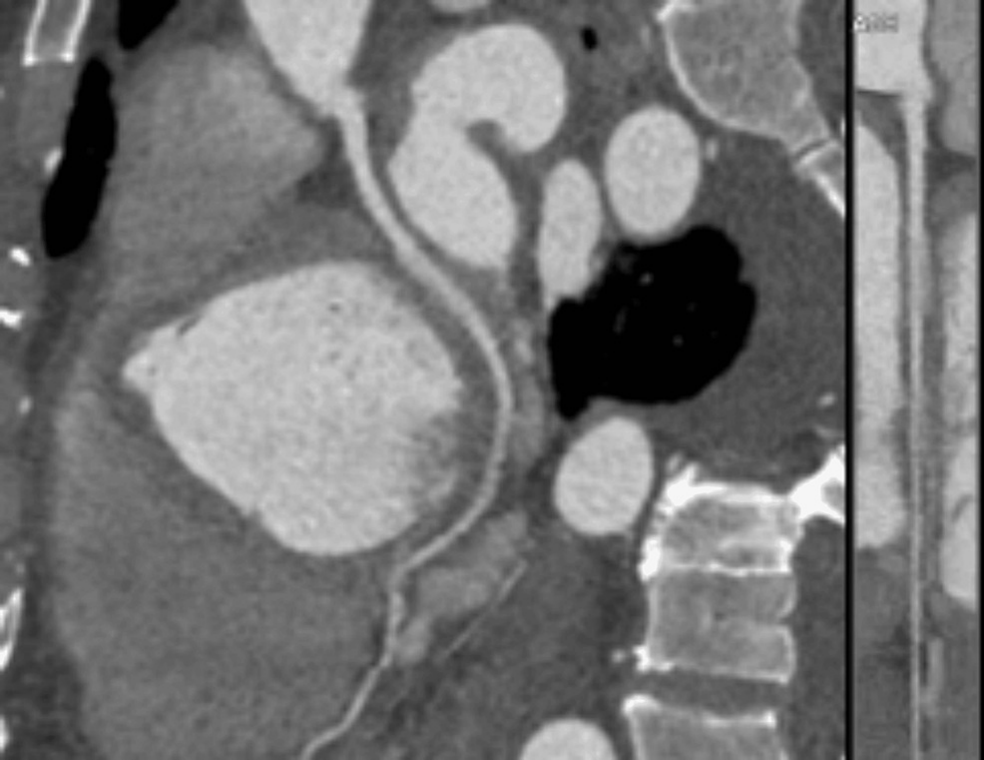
Left circumflex artery. Coronary computed tomography angiography shows the left circumflex artery being patent without any blockages or stenosis.
